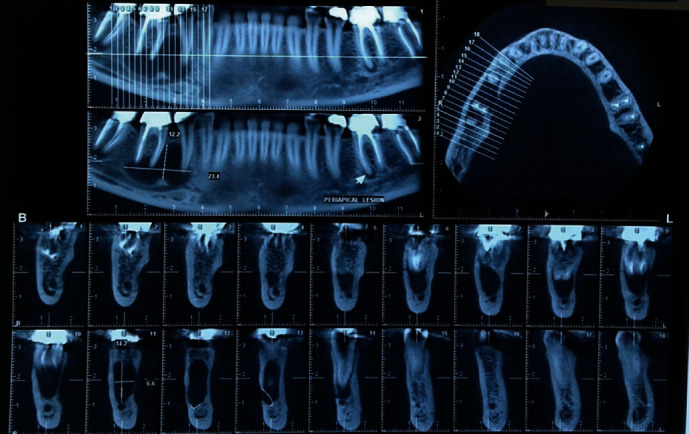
Initial CBCT obtained from the patient further revealing the extents of the lesion.
